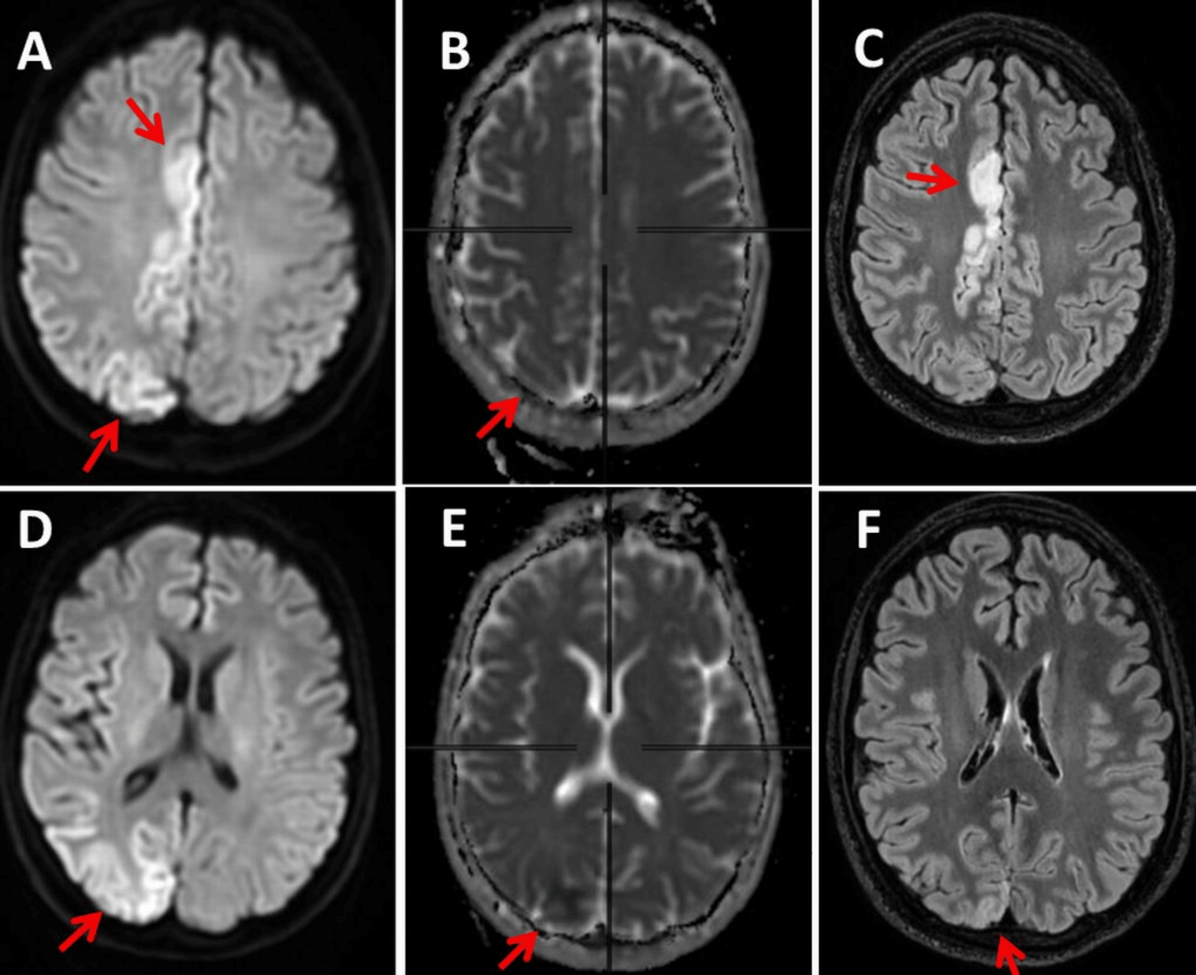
Cerebral MRI on hospital day 27, one day before extubation with regression of the SLL on DWI (panels A, D), ADC (panels B, E), and FLAIR (panels C, F) SLL: stroke-like lesion; DWI: diffusion-weighted imaging; ADC: apparent diffusion coefficient; FLAIR: fluid-attenuated inversion recovery
